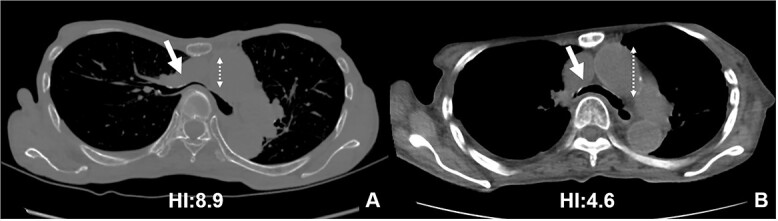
CT scans are used to monitor changes over time. In (A), chest CT findings of severe PE with stenosis of the right main bronchus. The trachea, which was centrally located on CT scan 8 years ago (2B), has shifted to the left now (2A). Additionally, the CT-HI worsened over his 8 years, increasing from 6.8 to 8.9.
